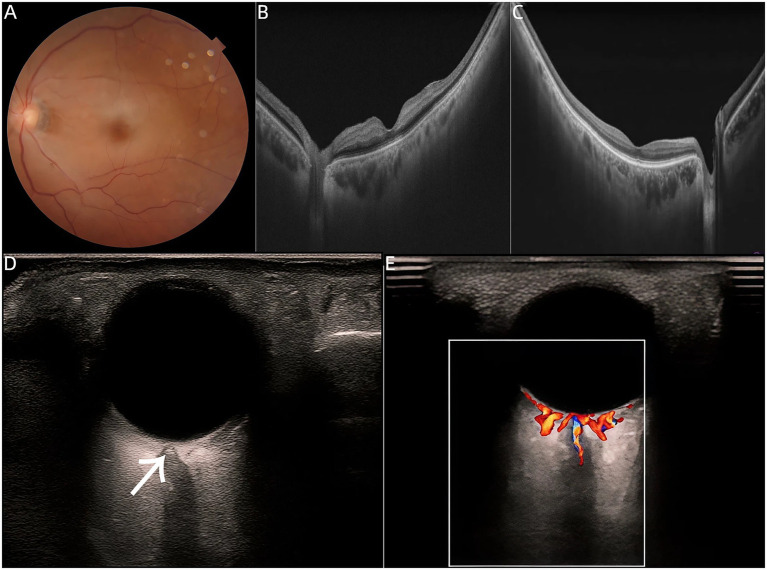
(A) Color fundus photography revealed perifoveal retinal whitening with a classic cherry-red spot at the foveola in the left eye. (B) OCT of the left eye demonstrated hyper-reflectivity confined to the inner nuclear layer. (C) OCT of the right eye showed normal retinal thickness and well-preserved laminar architecture across all retinal layers. (D) Ultrasound image showing a hyperechoic retrobulbar spot sign (RBSS) within the optic nerve in the right eye in Case 1 (arrow), measuring 1.4 × 1.3 mm. (E) Despite the presence of RBSS, ultrasound demonstrates abundant arterial blood flow in the right eye.
